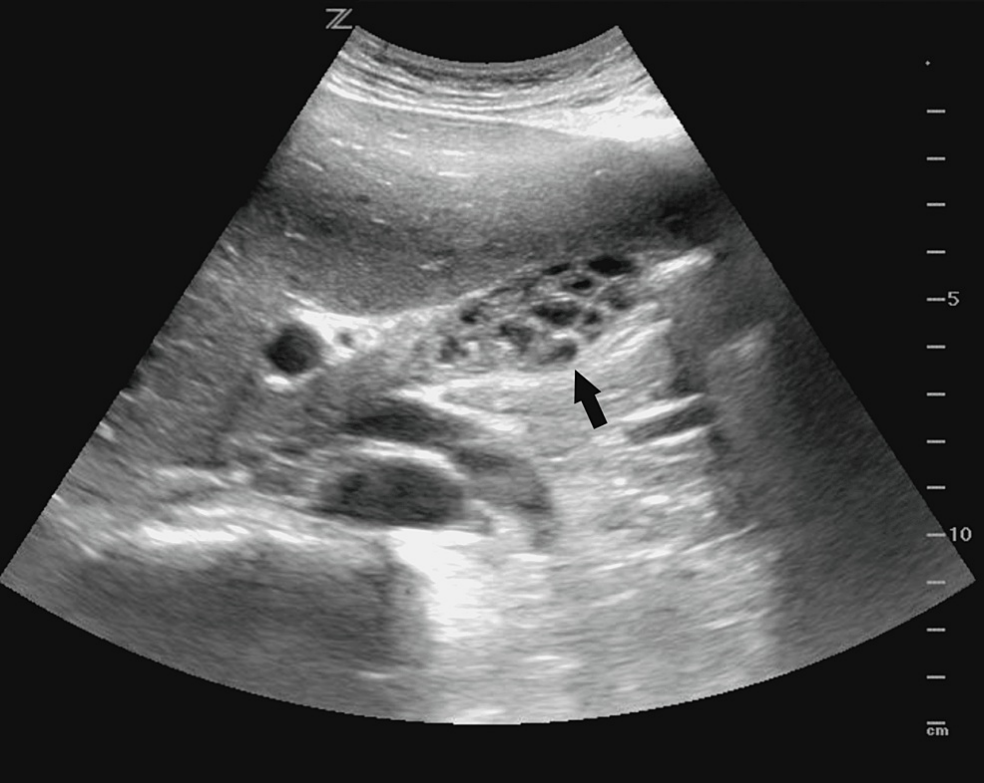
Multiseptated gallbladder (black arrow) seen on ultrasound taken in the emergency department. Multiple septations are noted on the point-of-care ultrasound performed initially in the emergency department.
